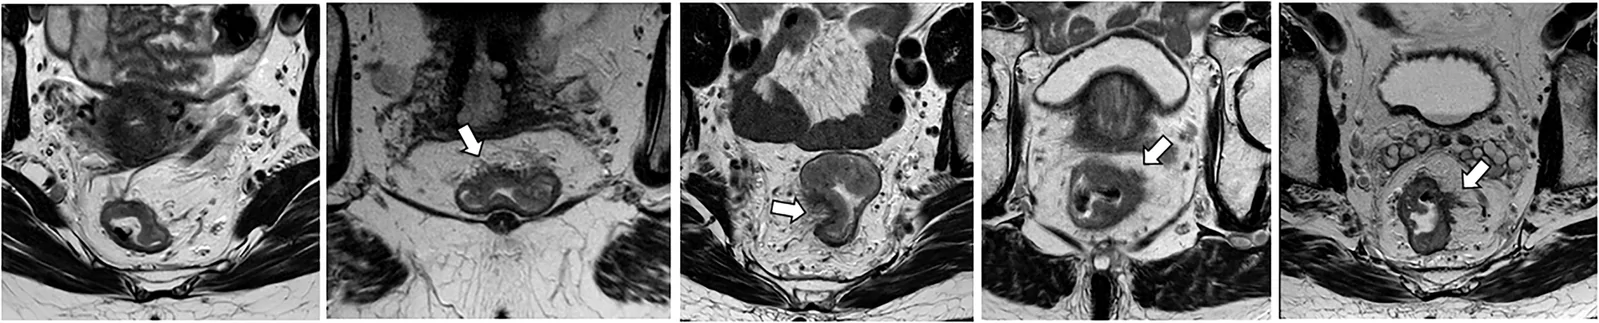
EMVI scoring system for MRI. Left to right: score 0—no EMVI; score 1—minimal extramural stranding, probably no EMVI (white arrow); score 2—stranding in proximity of vessels, no tumor signal, equivocal (white arrow); score 3—vessel expansion, probably present EMVI (white arrow); score 4—tumor signal, vessel expansion and irregular vessel contour, definitely present EMVI (white arrow). EMVI status is negative for scores 0–2 and positive for scores 3–4
